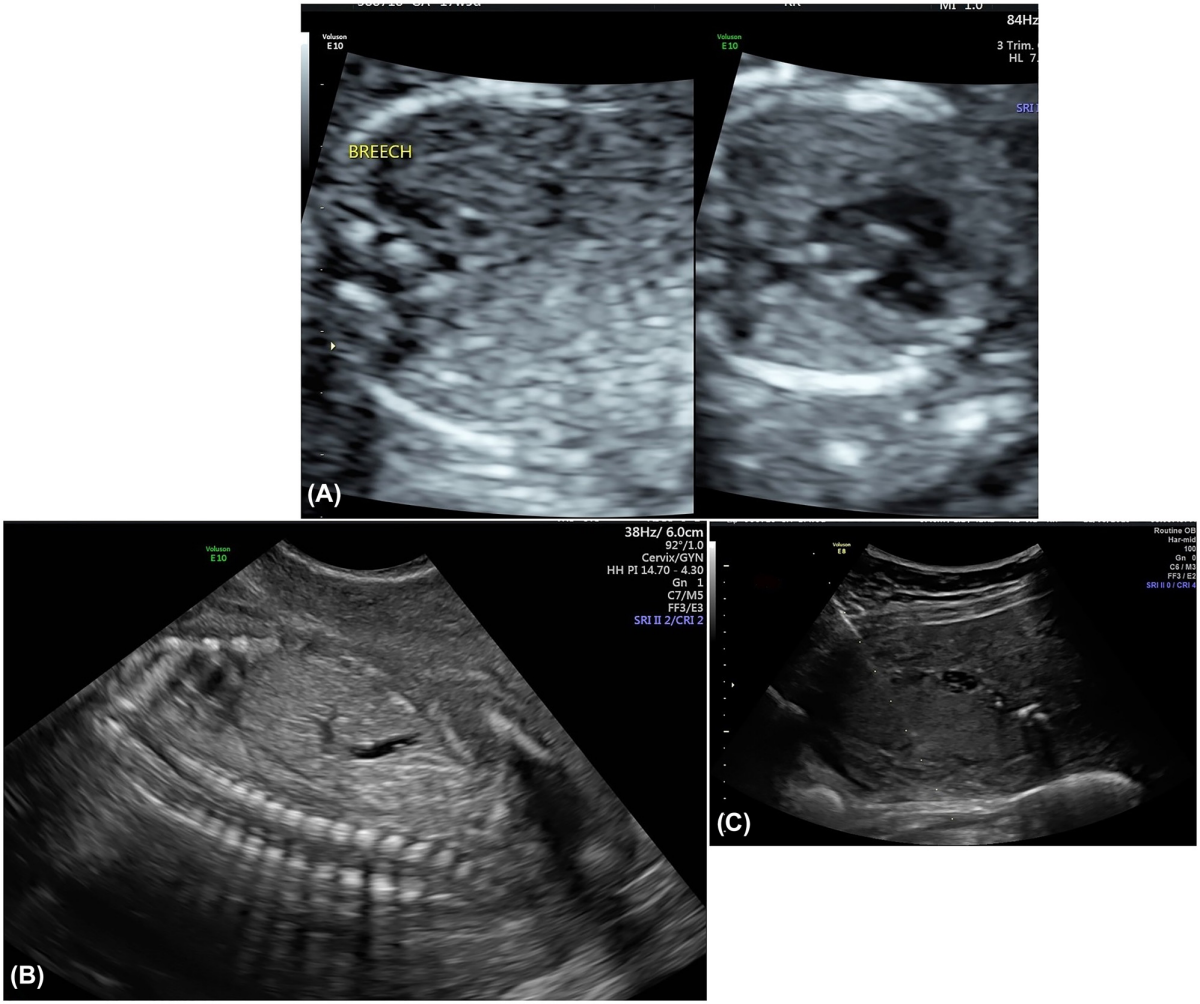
Ultrasound images of proband of family 2. (A) Transverse views of fetus that show empty renal fossa bilaterally and normally oriented heart. (B) Anhydramnios and absence of fluid filled stomach and bladder. (C) Placental biopsy.
